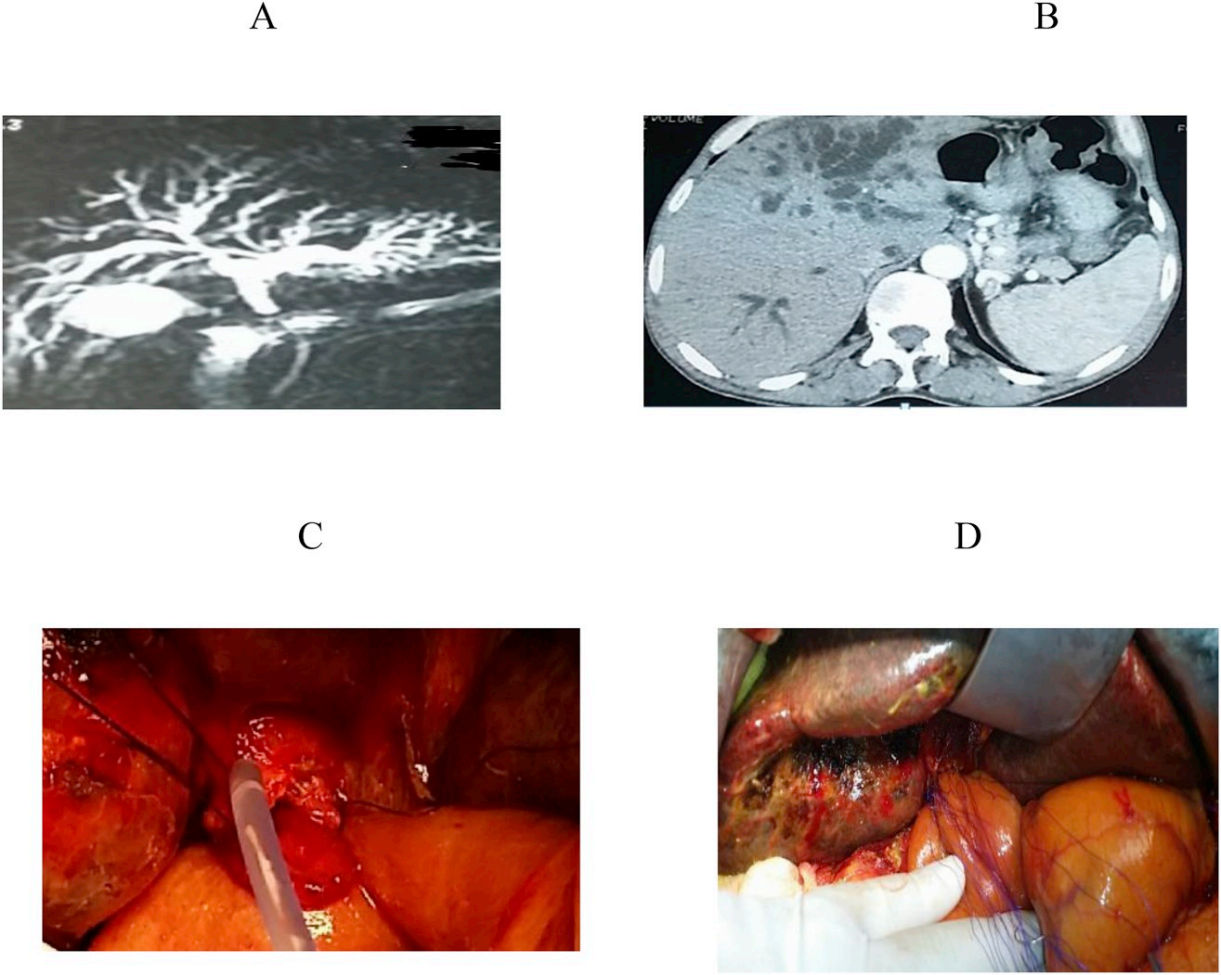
A, B: MRCP and CT showing type E1 BI, C, D: RY HJ without stent.
